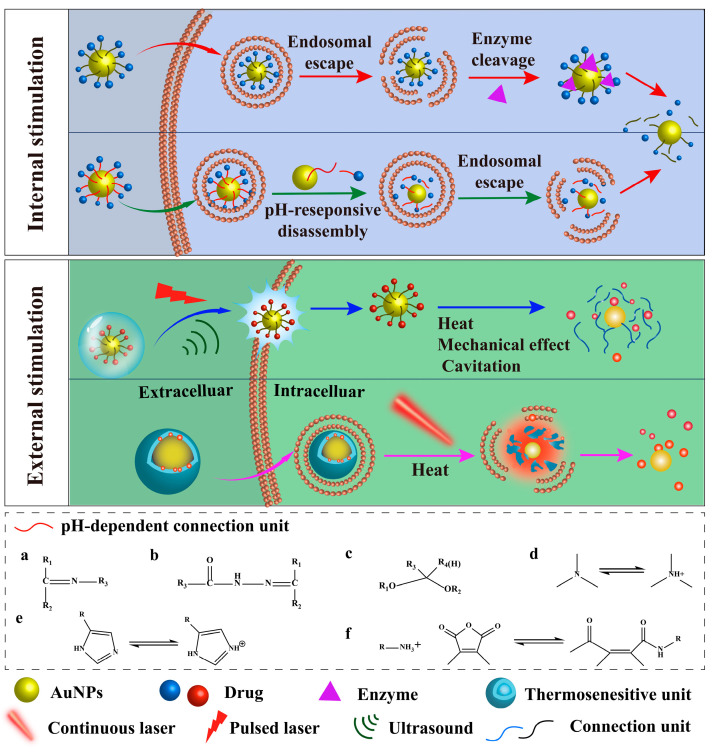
Strategies of drug release mediated by AuNPs as carriers primarily include internal and external stimulation. The internal stimulation-mediated drug release mainly includes (I) enzyme-stimulated and (II) pH-responsive drug release. The PH-responsive drug release is mediated by pH-sensitive connection units, commonly including (a) Schiff base compounds, (b) acyl-brown bonds [168,169], (c) acetal/ketone structures [170], (d) tertiary amino protonation [171], (e) the reaction of amino groups with 2,3-dimethylmaleic anhydride [172], and (f) the protonation of imidazole groups [173]. The external stimulation is based on the ultrasound, pulsed laser, and continuous laser external stimulation of AuNPs.
